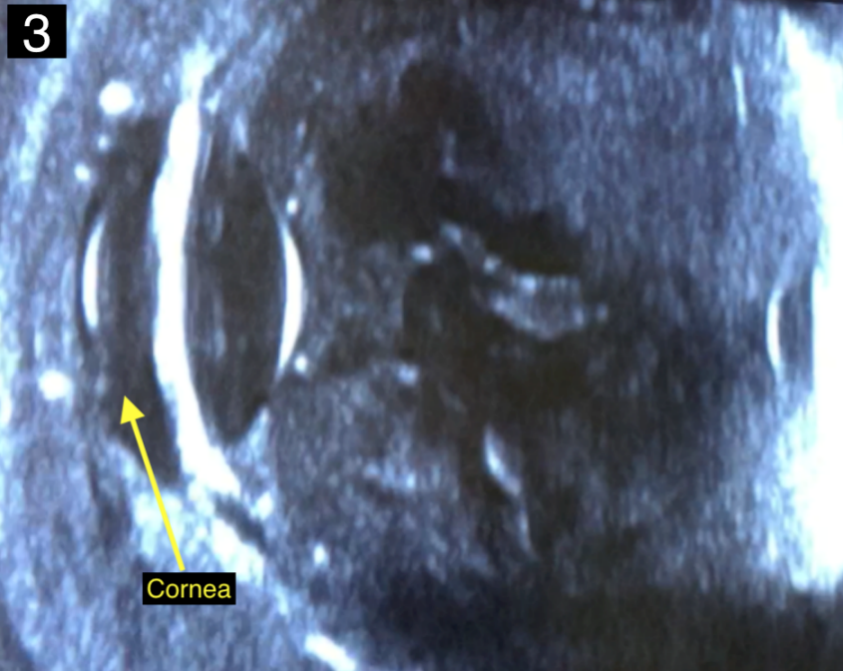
Ultrasound image of the right eye demonstrating dense hyperechoic cellular debris within the vitreous cavity suggestive of vitritis
